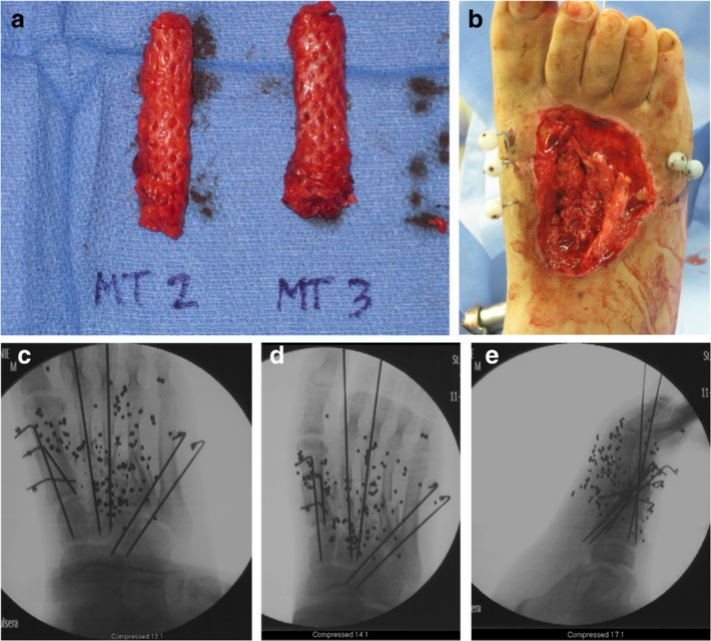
Intra-operative photographs (a-b) and radiographs (c-e) of the right foot. Final fascial graft encasing autograft, cancellous allograft, and BMP-2 (A) for each metatarsal. Figure 4b demonstrates *in situ* placement of the grafts, with corresponding intra-operative fluoroscopic anteroposterior (AP), oblique, and lateral views (c-e).
